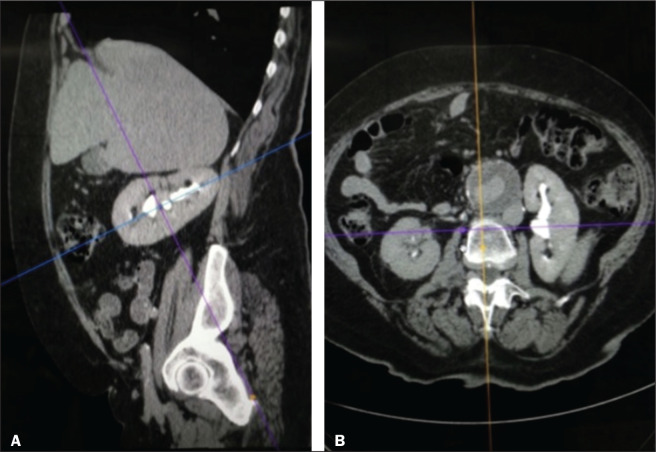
Sagittal and axial CT reconstructions of the kidney (A and B, respectively), based on the main axis of the organ. Note how, in the sagittal view, the kidney is rotated in relation to the spine.
